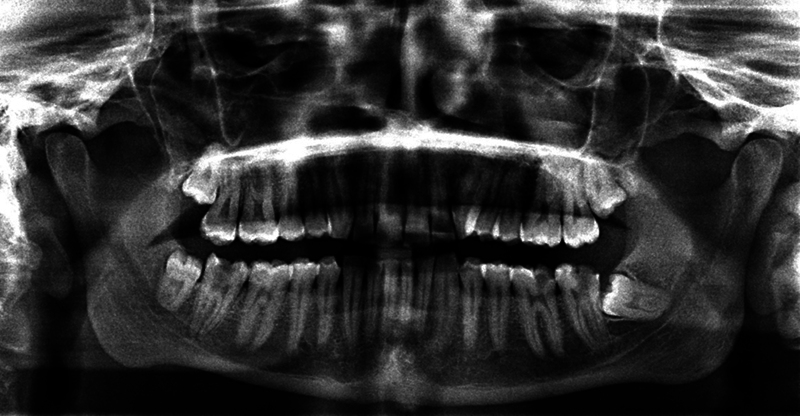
Orthopantomogram showing bilateral taurodonts in the maxillary teeth.
